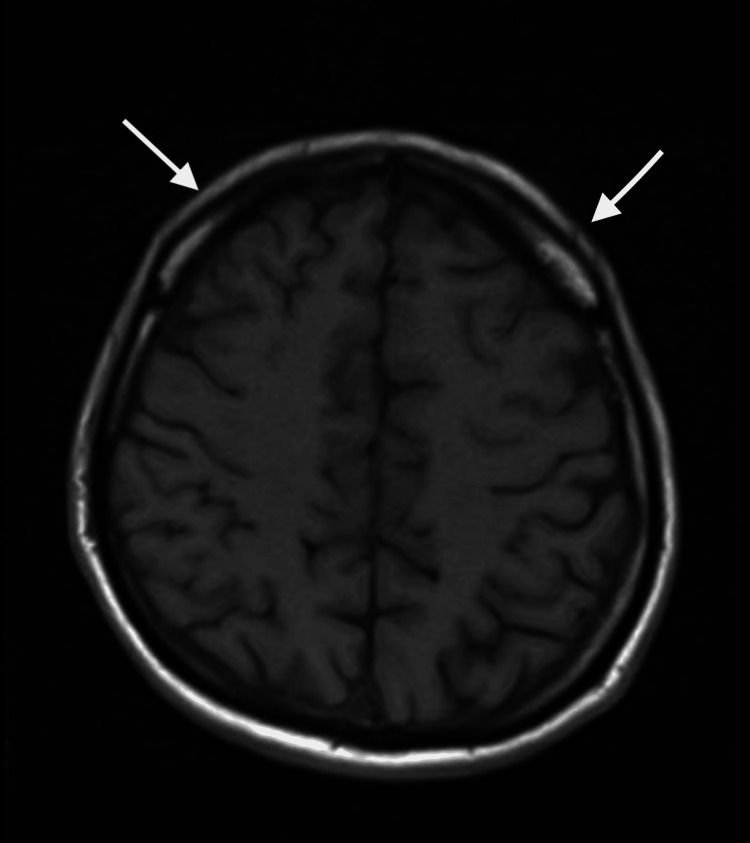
MRI of the brain showing subarachnoid punctate hyperintensity
